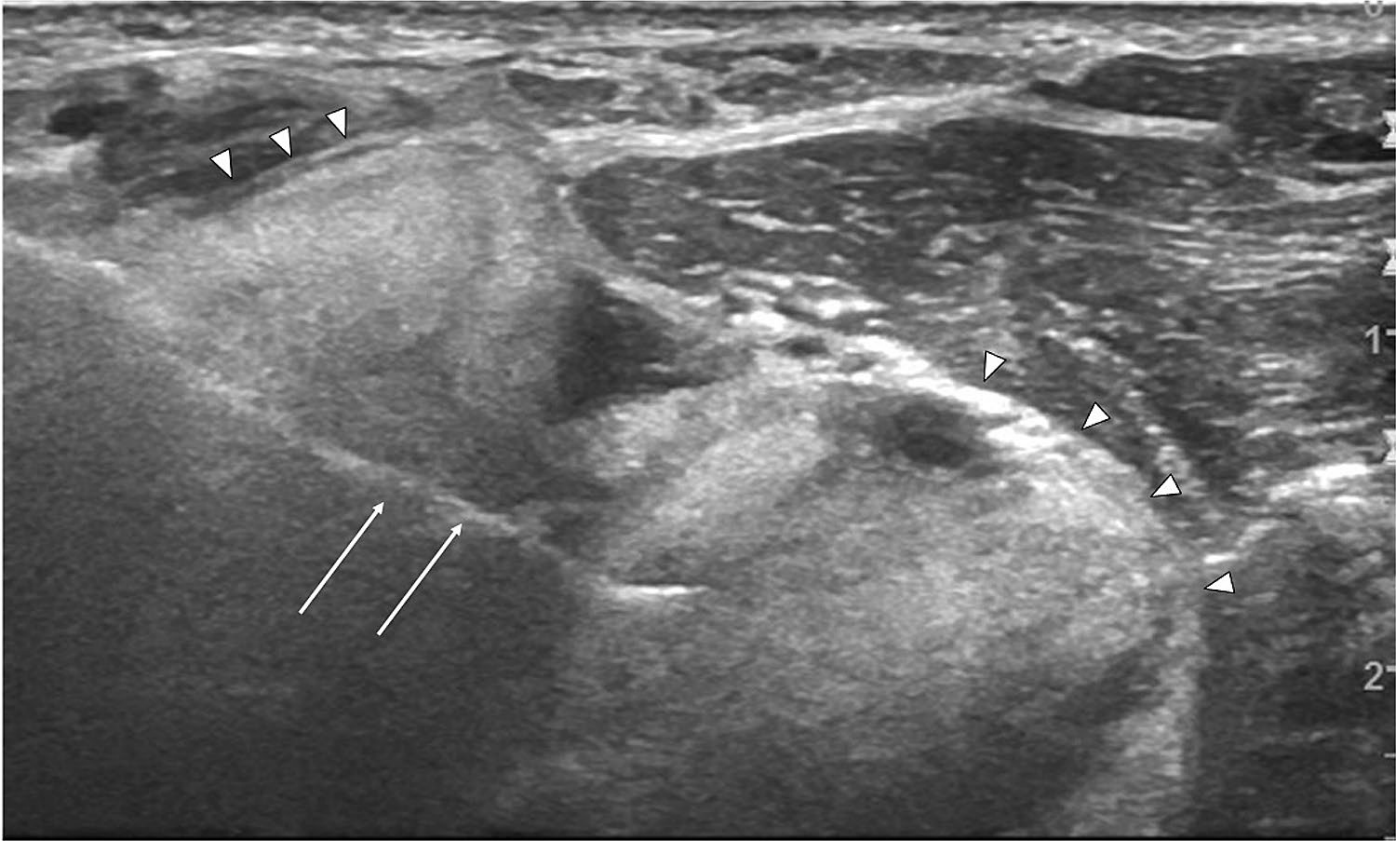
65-year-old man with a PMT of the right elbow. Ultrasound of the proximal forearm shows a large extraosseous component (arrow heads) which was not apparent on plain radiograph. Biopsy needle (arrows)
